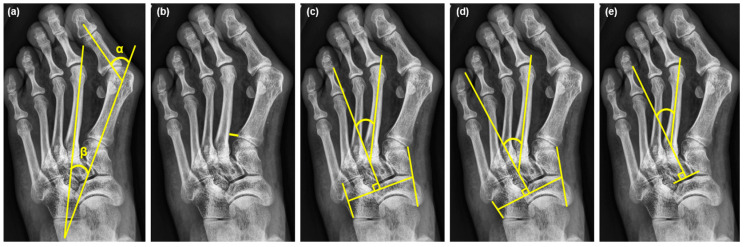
The radiographic measurements. (a) α: hallux valgus angle (HVA), β: intermetatarsal angle (IMA); (b) the distance between the first and second metatarsal bases (M1-2 distance); (c) Sgarlato’s MAA (MAA4); (d) modified Sgarlato’s MAA (MAA5); (e) modified Engel’s MAA. These weight-bearing foot radiographs of a 62-year-old female hallux valgus patient with metatarsus adductus provided the following measurements: HVA: 51°, IMA: 13°, MAA4: 28.05°, MMA5: 34.28°, modified Engel’s MAA: 33.07°, and M1-2 distance: 4.2 mm.
